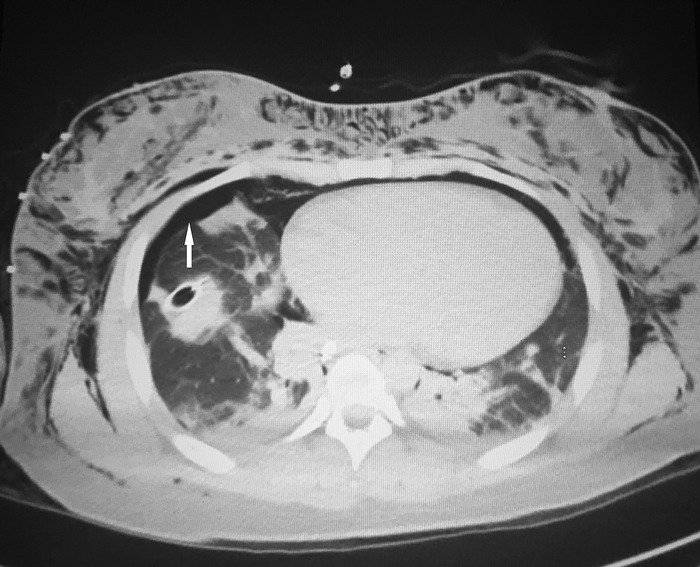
Postoperative computed tomography of the chest shows subcutaneous air and right-sided pneumothorax (white arrow).
